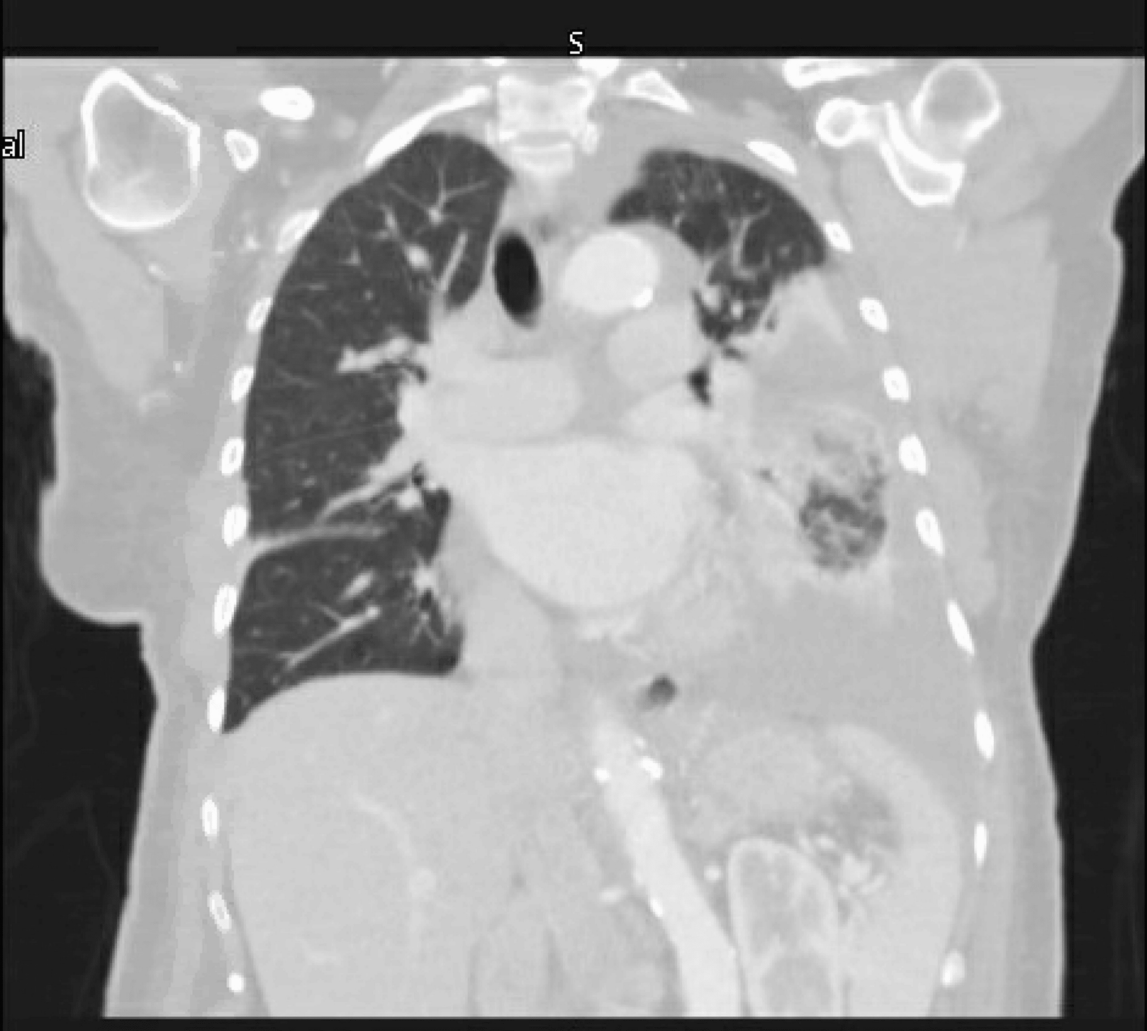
Computed tomography scan of the chest without contrast There is a mass opacification with bronchial obstruction in the left lower lobe
